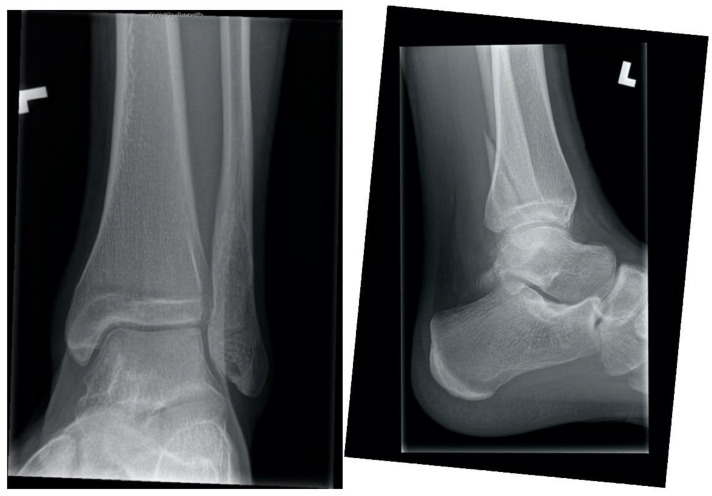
Twelve-week ankle radiographs demonstrating no radiographic union after fibula fracture in a pediatric electronic cigarette user.
